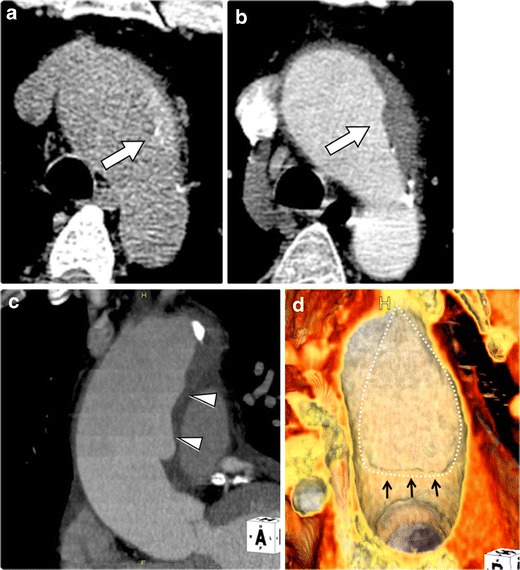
Limited intimal tear with intramural haemorrhagic content in a 48-year-old male. a Non-contrast CT demonstrates the aortic wall thickening with haemorrhagic content (*arrow*) at the proximal aortic arch. b Contrast-enhanced CTA demonstrates the aortic wall thickening (*arrow*) without clear intimal-medial flap or false lumen visualized; this was initially diagnosed as traditional IMH. c Slab-MIP image demonstrates the ‘eccentric one-sided bulge’ of the aortic wall along the ascending aorta (*arrowheads*). d 3D-VR luminal image right in front of the bulge shows a teardrop-shaped intimal tear (*dotted line*) with a localized intimal flap (*black arrows*) at the inferior border of the intimal tear
